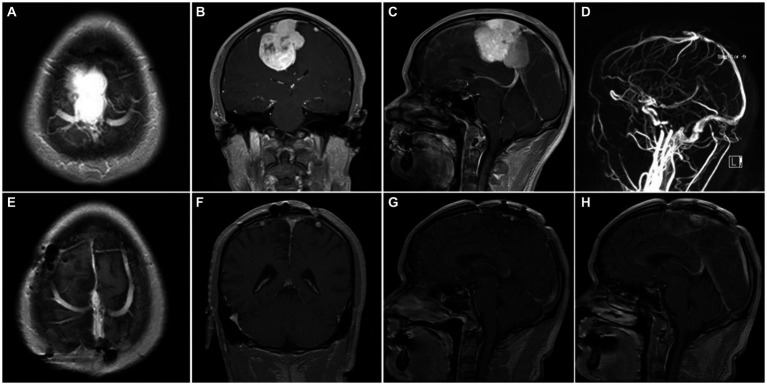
Representative case 4, type III (complete sinus obstruction). (A–C) preoperative enhanced MRI images. (D) preoperative MRV images. (E–H) Postoperative enhanced MRI images. Intraoperative sinus ligation and division without sinus reconstruction.
